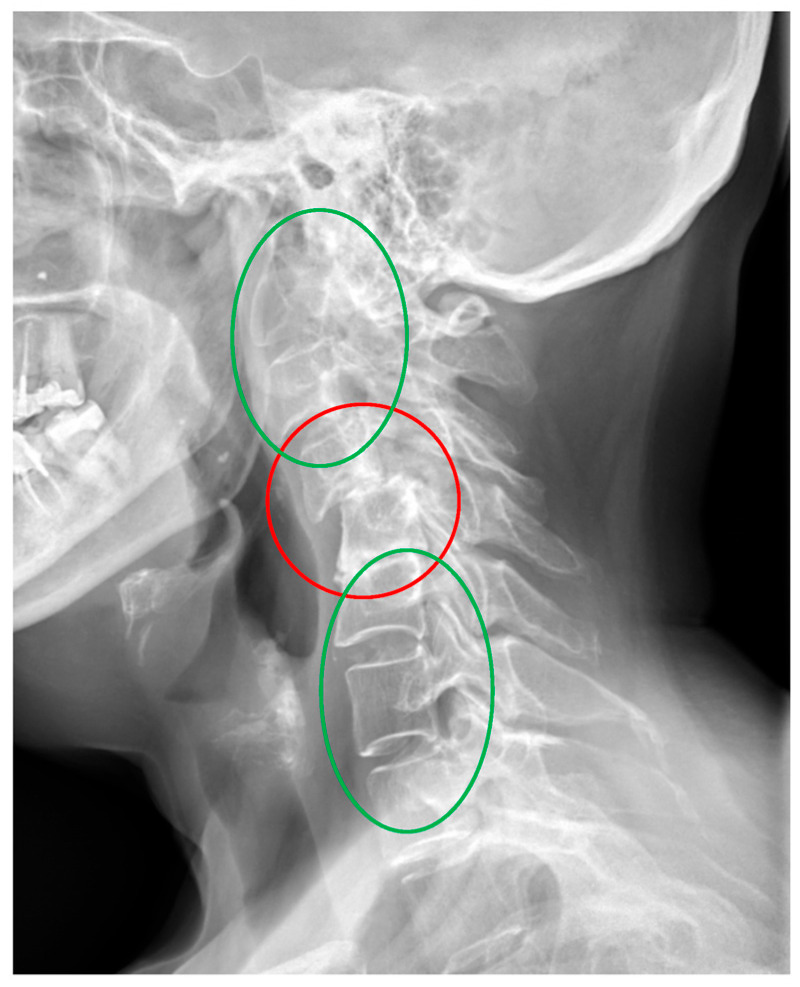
An X-ray of the cervical spine (lateral view) of a 54-year-old female patient with RA and SAS instability. C3–C4, C4–C5 instability (red). Cervical deformity with lordosis below C4–C5 segment (green) and lordosis above C4–C5 segment (green).
